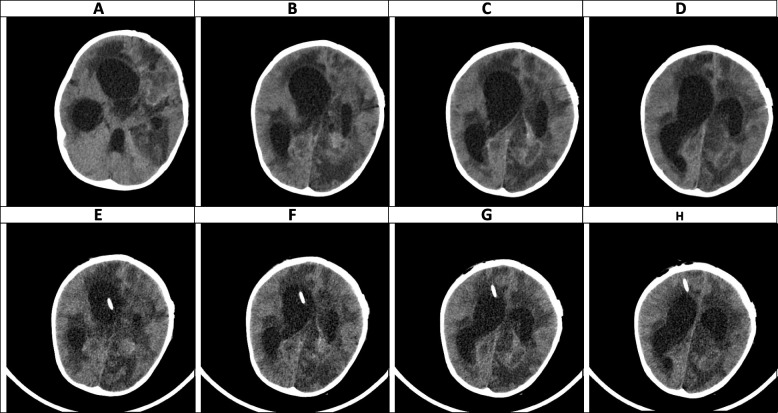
(the Brain CT SCAN without contrast, day 25). A-D (before EVD insertion) and E–H (after EVD insertion). A-D Sign of severe hydrocephalus associated with some shift of midline structures seen to the left side. Signs of multiple hypodense lesions seen at the different parts of the brain parenchyma which is due to mentioned abscess formations. E–H Except for mentioned findings in the previous CT SCAN, shadow of a EVD is seen traversing the right frontal lobe and entering the right lateral ventricle, however, the hydrocephalus is persisted which can be due to doing CT SCAN just after EVD insertion rather than EVD malfunction
